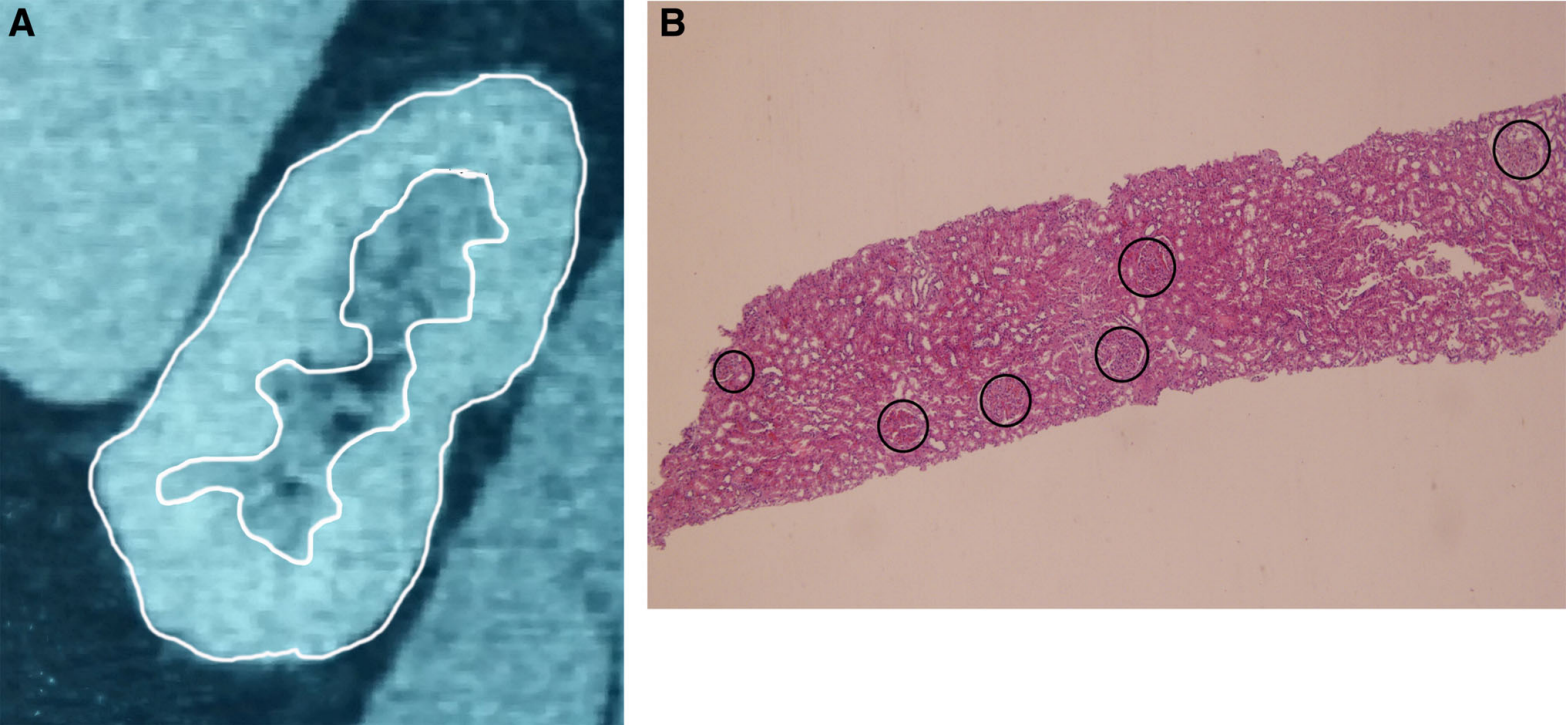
Enhanced CT and renal biopsy. (A). CT of a kidney with the cortex outlined in white. Serial reconstruction then allows an estimate of total cortical volume. (B). A renal biopsy 8.0 × 1.8 mm contains six glomeruli for a glomerular density of 0.42/mm^2^. Mean glomerular area for the biopsy is measured, and volume and density are estimated in three dimensions by Weibel and Gomez formulae (1962)
